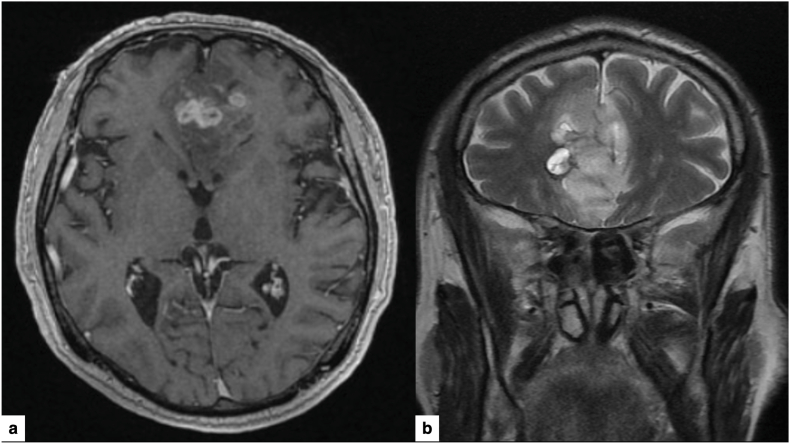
Preoperative MRI. (a) Axial T1-weighted post-contrast image showing a bilateral frontal “butterfly” lesion crossing the corpus callosum. (b) Coronal T2-weighted image demonstrating the infiltrative nature and extent of the lesion.
